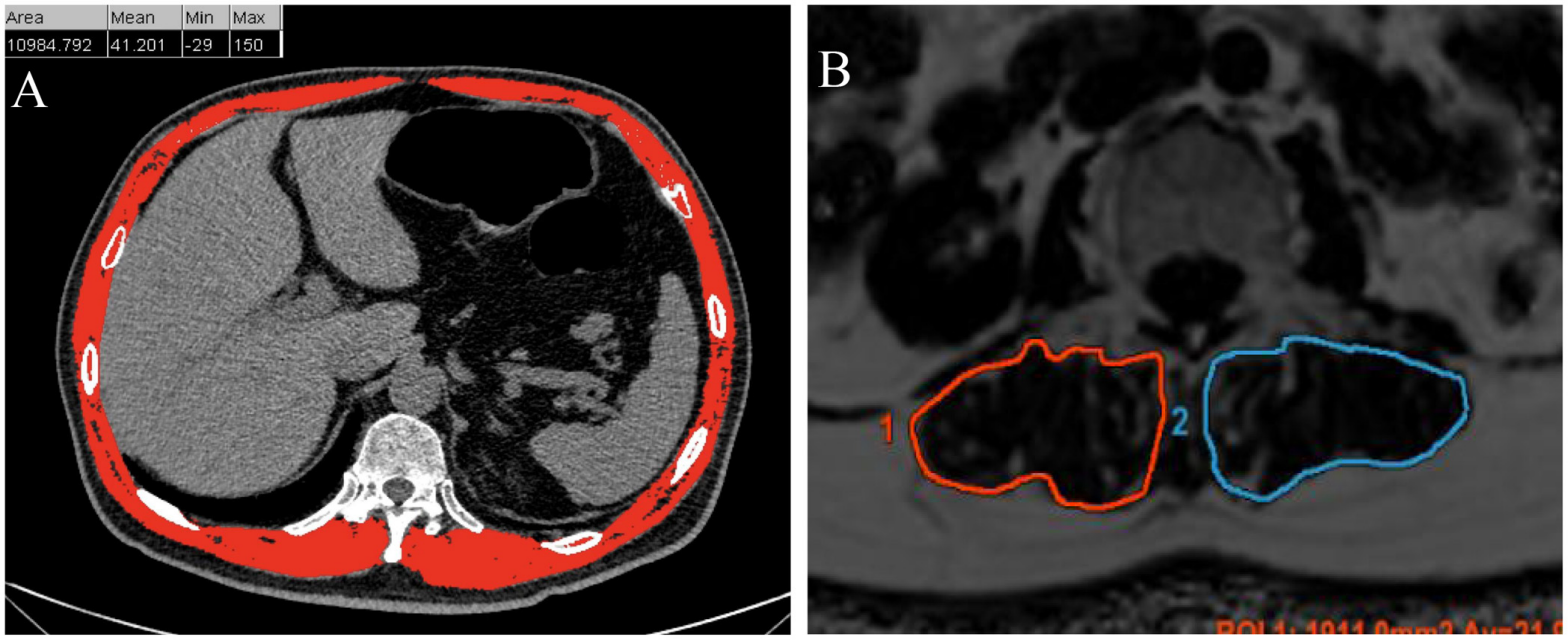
The evaluation of muscle fat infiltration was executed through computed tomography (CT) (A) and quantitative magnetic resonance imaging (MRI) (B) independently. The CT attenuation of all muscles at the middle layer of T12 was ascertained. The fat fraction of the multifidus and erector spinae muscle at the level of the third lumbar vertebra was determined using the MRI IDEAL-IQ sequence.
